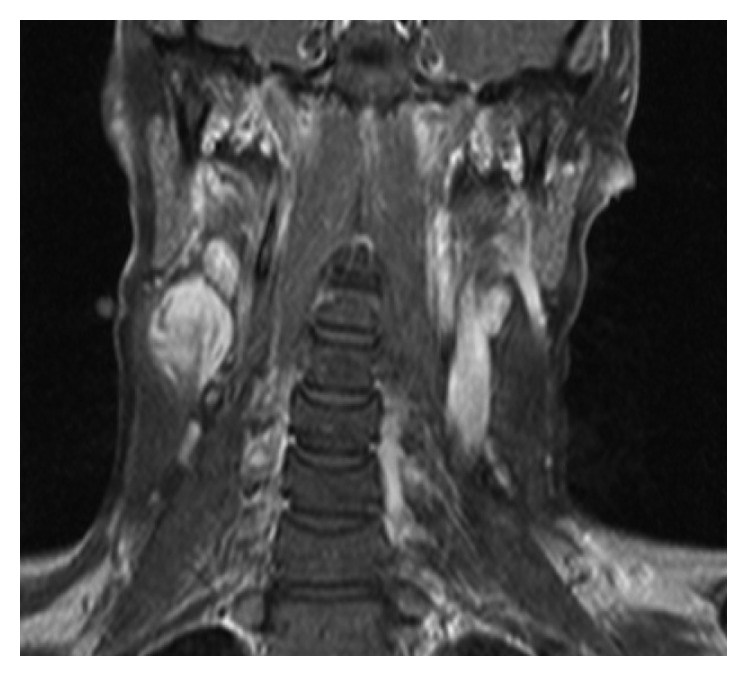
Coronal T1-weighted fast spin-echo (T1FSE) MRI depicting the tumor as an enhancing mass at the cephalic part of the right sternocleidomastoid muscle.
